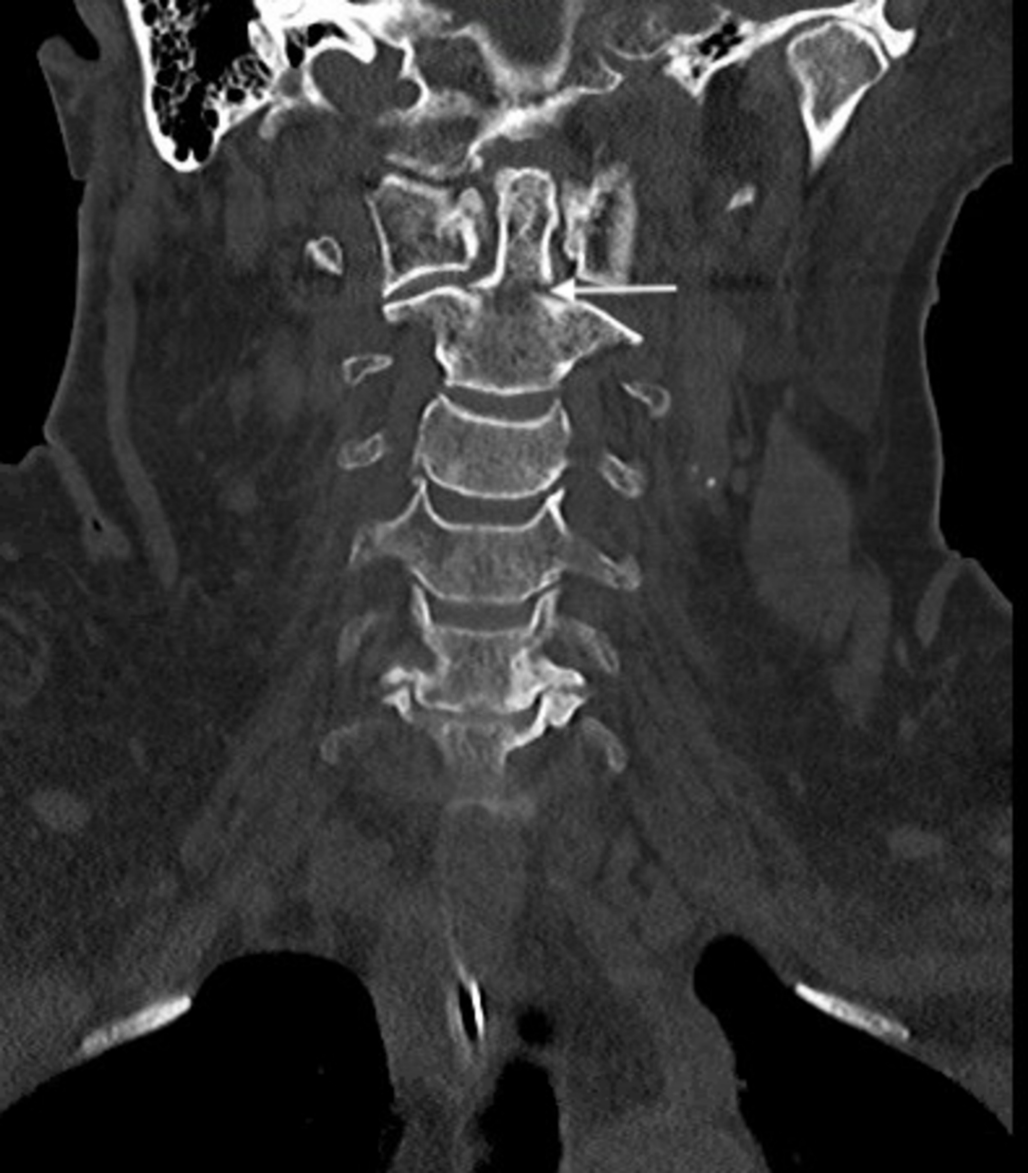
Coronal CT of the cervical spine with type II odontoid fracture. Coronal non-contrast CT bone window of type II odontoid fracture.
